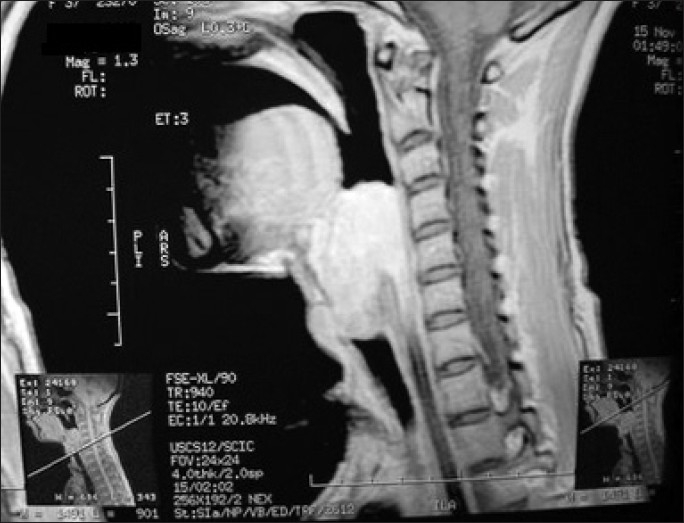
Computed tomography (CT) scan of neck revealing enhancing soft tissue mass starting below the vocal cord extended into the oropharynx (Sagital View)
